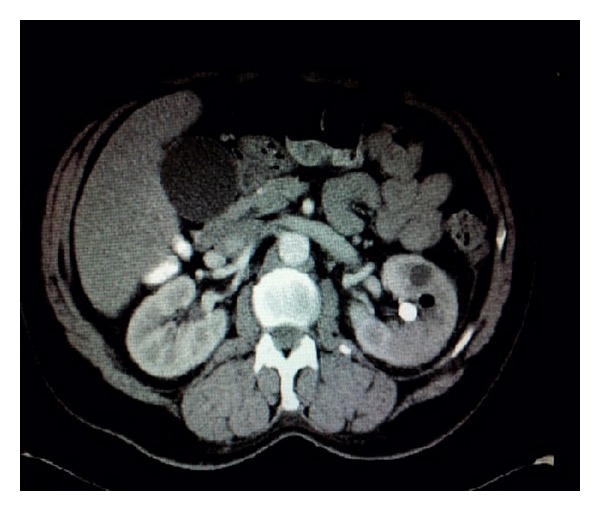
CT scan of the abdomen and pelvis showing left renal calculi with foci or air in the renal parenchyma.
